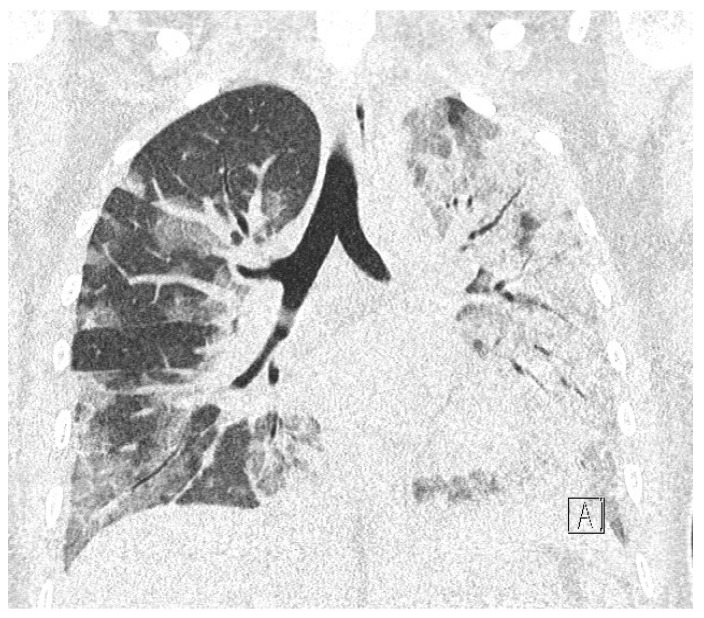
Chest Computed Tomography (HRCT).
